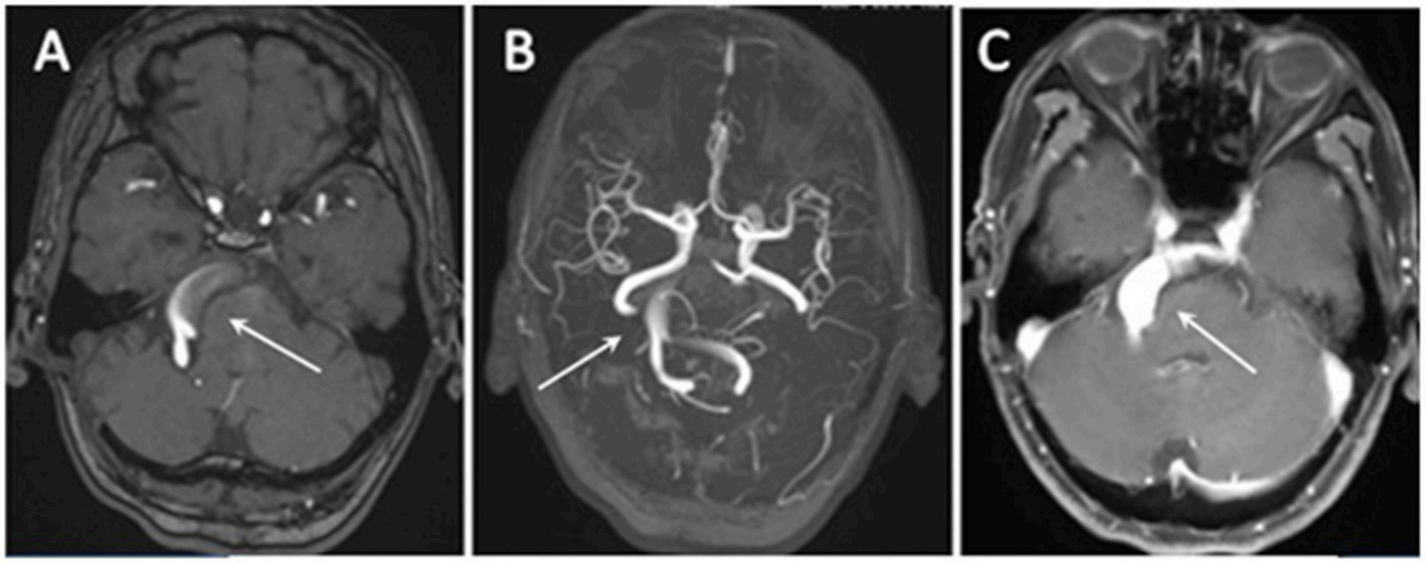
(A,B) 3D time-of-flight magnetic resonance angiography (TOF-MRA) revealed a dilated, tortuous, and elongated vertebral artery and basilar artery (arrow). (C) The contrast-enhanced MRI revealed a significant expansion of the basilar artery (arrow).
